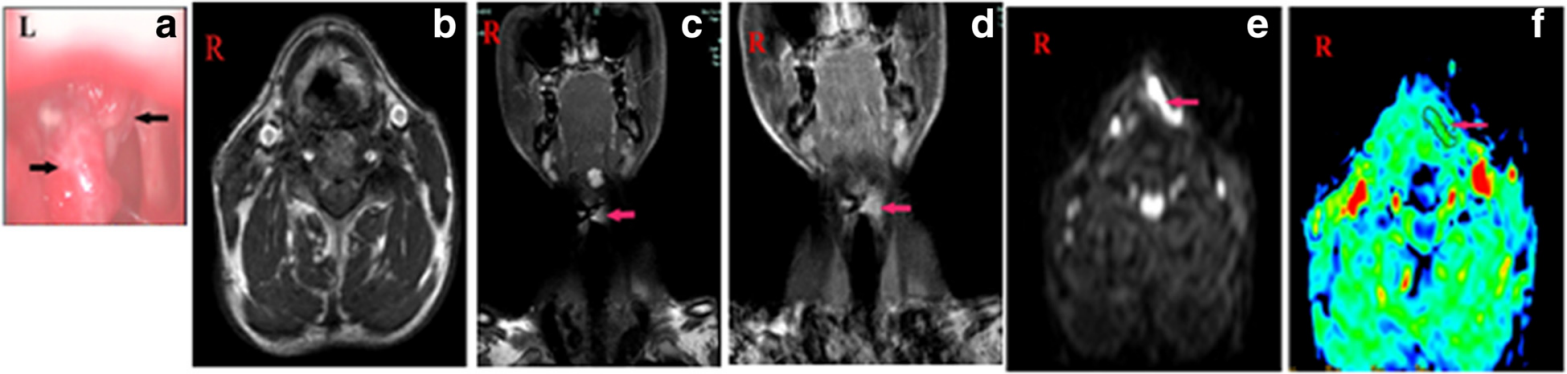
Laryngostroscopy and MRI of Case 8. a) Laryngostroscopy showinga red smooth mass in the left false vocal cord involving in the left vocal cord, anterior commission, and left aryepiglottic fold. An MRI scan revealed that there was a mass in the left false vocal cord that also involved the left vocal cord. b) TheT1-weighted signals and c) the T2-weighted signals were hyperintense, d) and the contrast-enhanced T1-weighted MRI images show strong enhancement. e) DWI suggested hyperintense lesions were in the left false vocal cord (b = 1000s/mm^2^). f) The corresponding ADC map reveals a hypointense mass of lesions in the left false vocal cord (ADC = 0.793 × 10^-3^ mm^2^/s).
